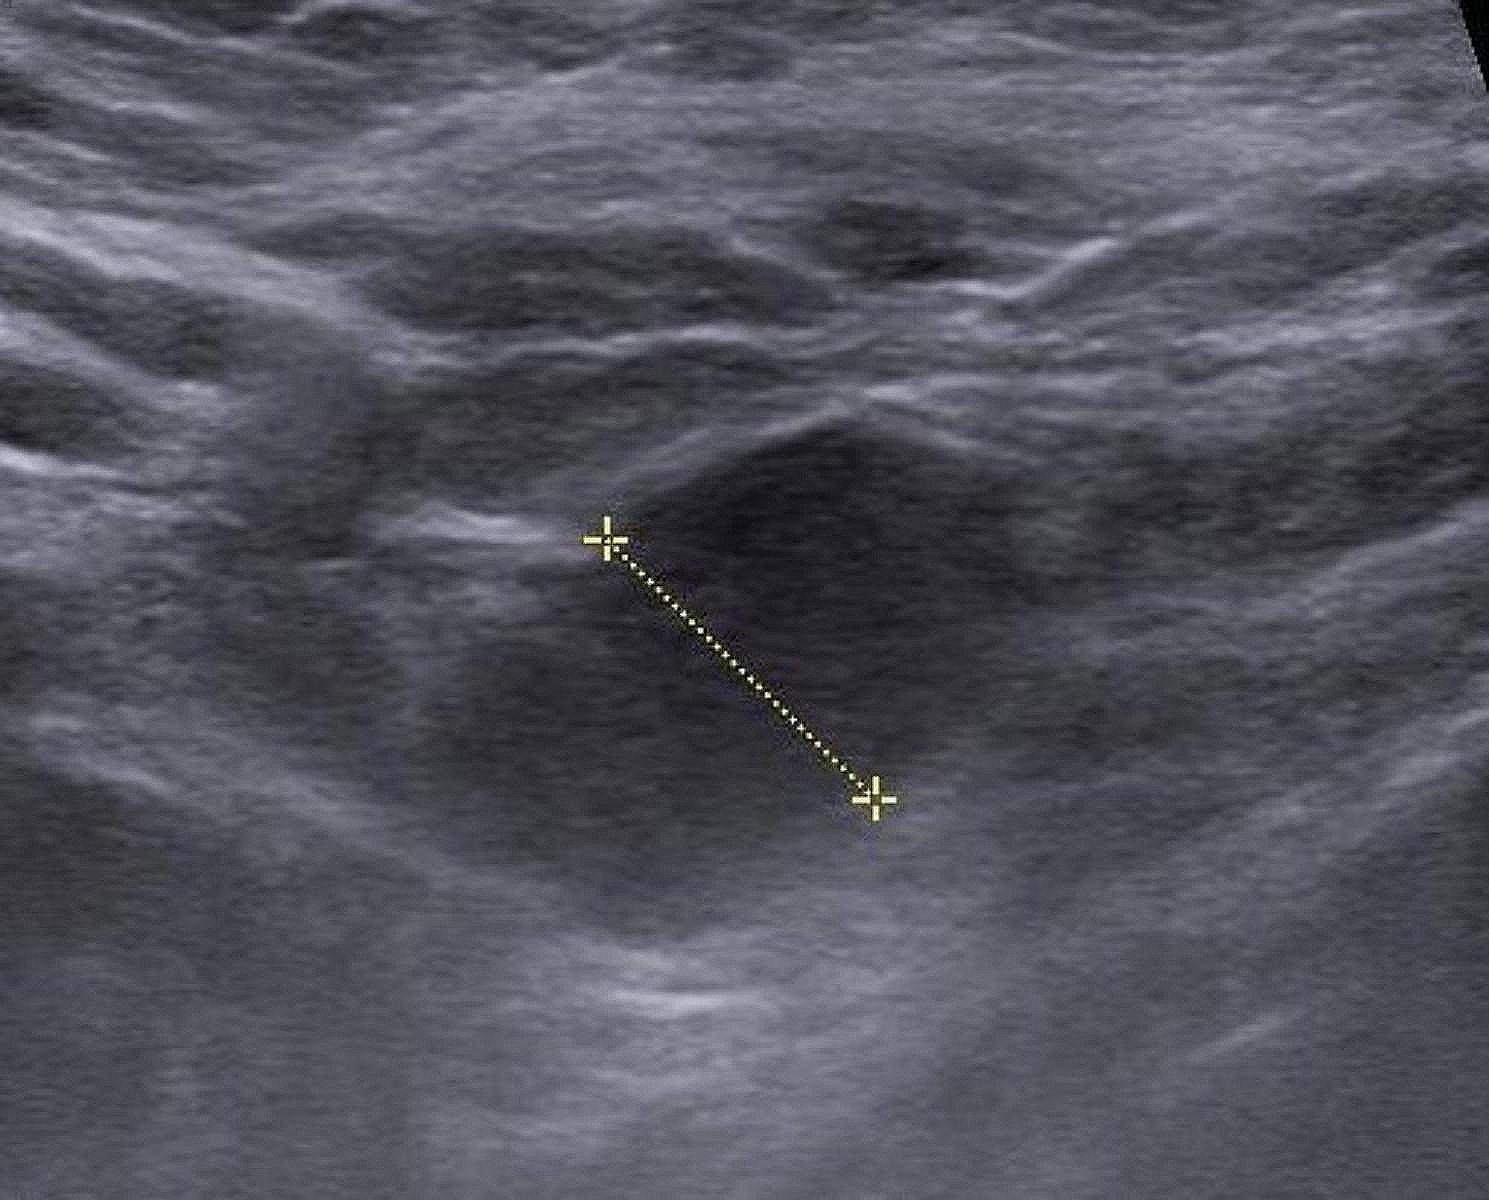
Ultrasonography of the axillary mass
